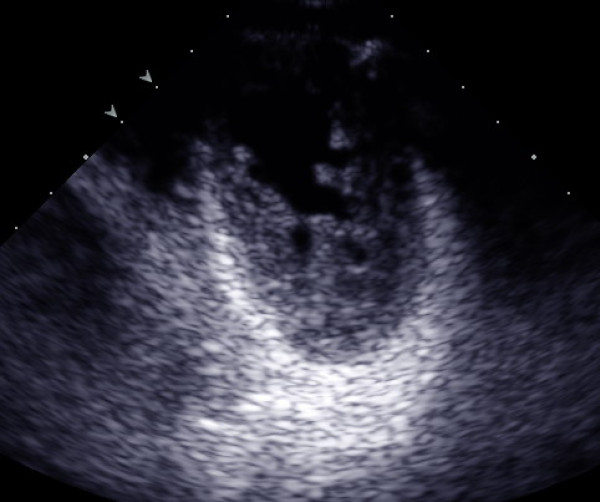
Apical short-axis view shows numerous, excessively prominent trabeculations associated with intertrabecular recesses by two-dimensional echocardiography.
